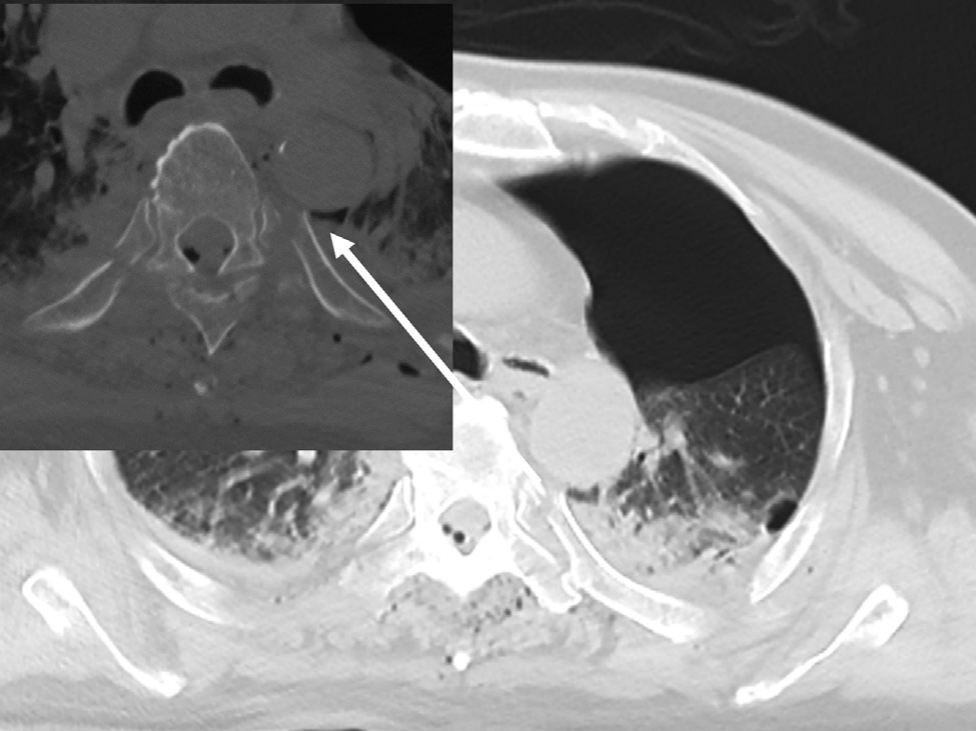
Thoracic computed tomography (CT) on arrival in case 2. CT shows the spiculated end of the fifth fractured rib adjacent to the descending aorta (arrow).
